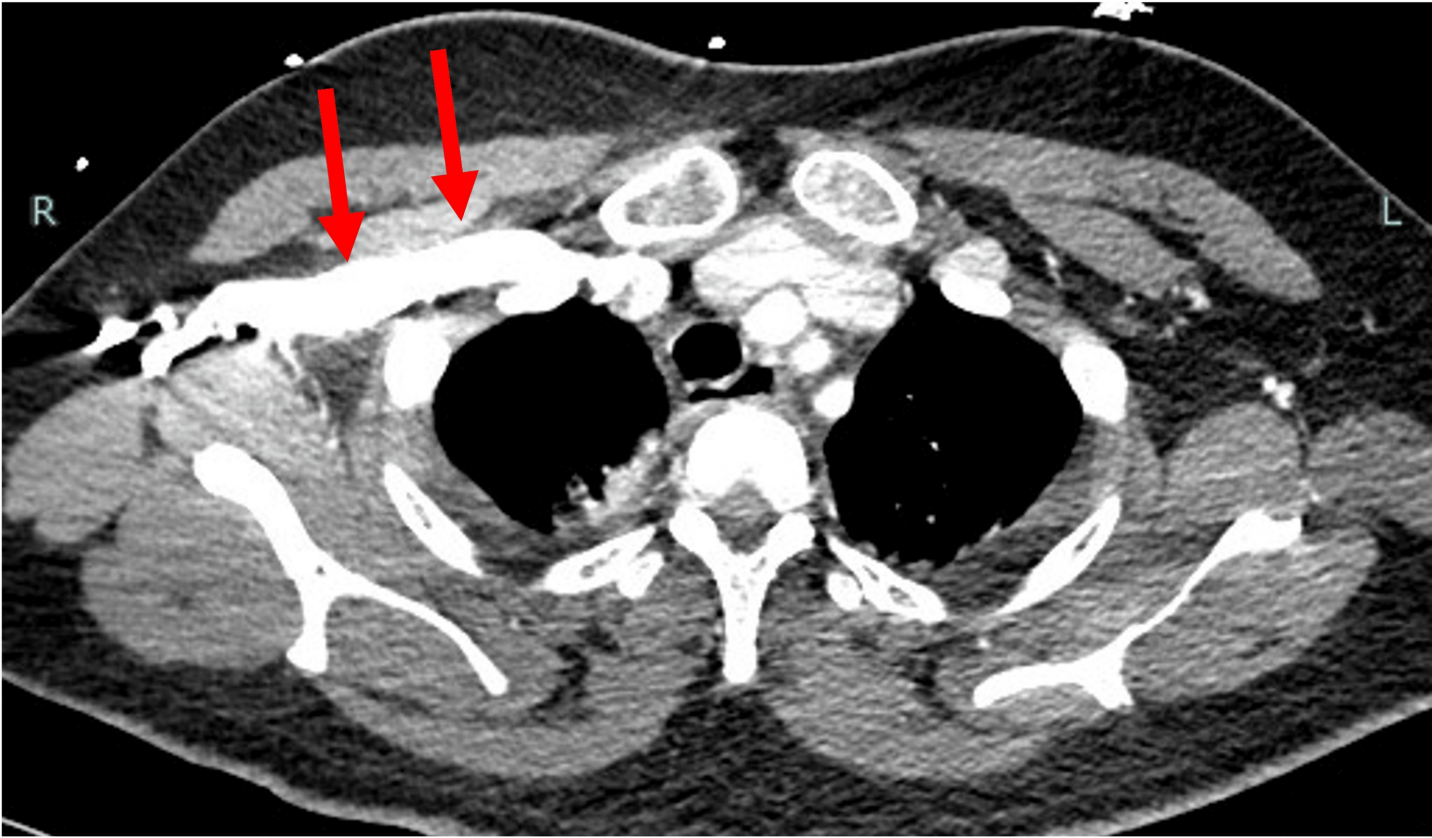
CT image at cardiopulmonary arrest. Contrast is highly stagnant in the brachiocephalic vein.
